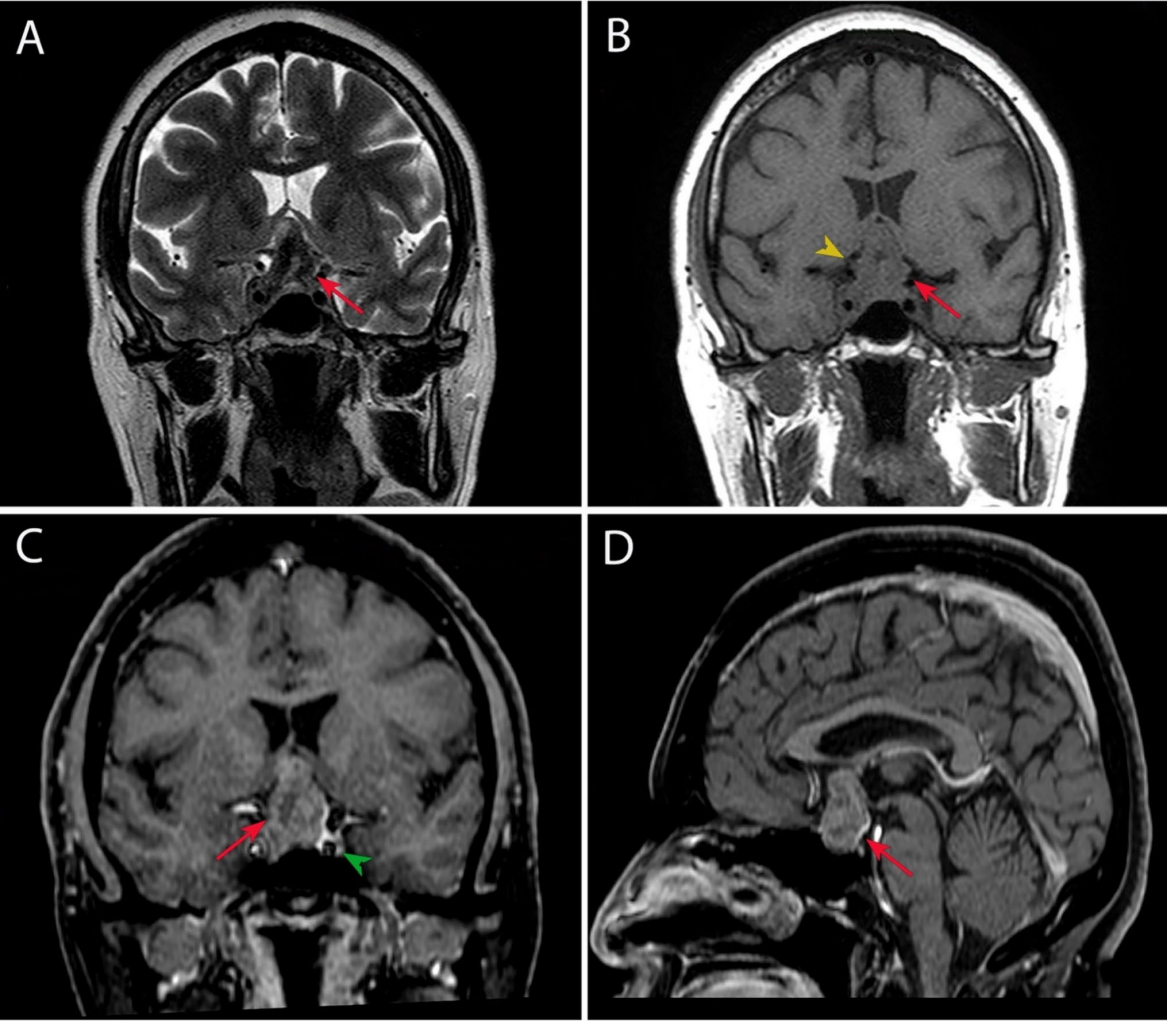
Radiological features of a heterogeneous space-occupying mass in different MRI sequences. A T2 coronal: heterogeneous space occupancy mass with hypointense areas in its periphery and isointense center (red arrow). B T1 simple coronal: an isointense space-occupying mass of irregular and undefined outline (red arrow) partially surrounds the right cavernous sinus with compression (yellow arrowhead) and dorsal displacement of the optic chiasm. C T1 coronal FAT SAT with contrast: moderate reinforcement is seen with central areas with necrosis (red arrow). The internal carotids are permeable (green arrowhead). D T1, sagittal with gadolinium: peripheral reinforcement is seen with central necrosis (red arrow); optic chiasm is not identified
